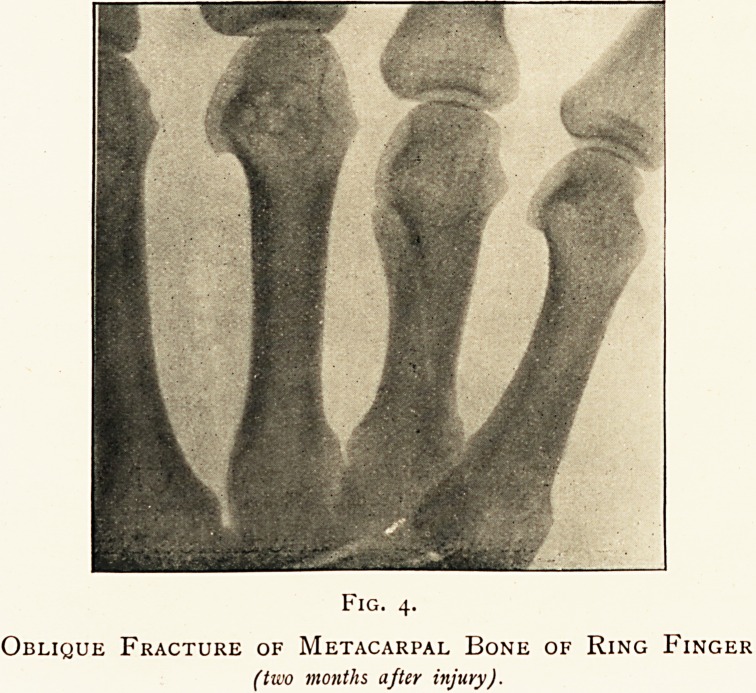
# A Simple Form of Influence Machine for X-Ray Work
